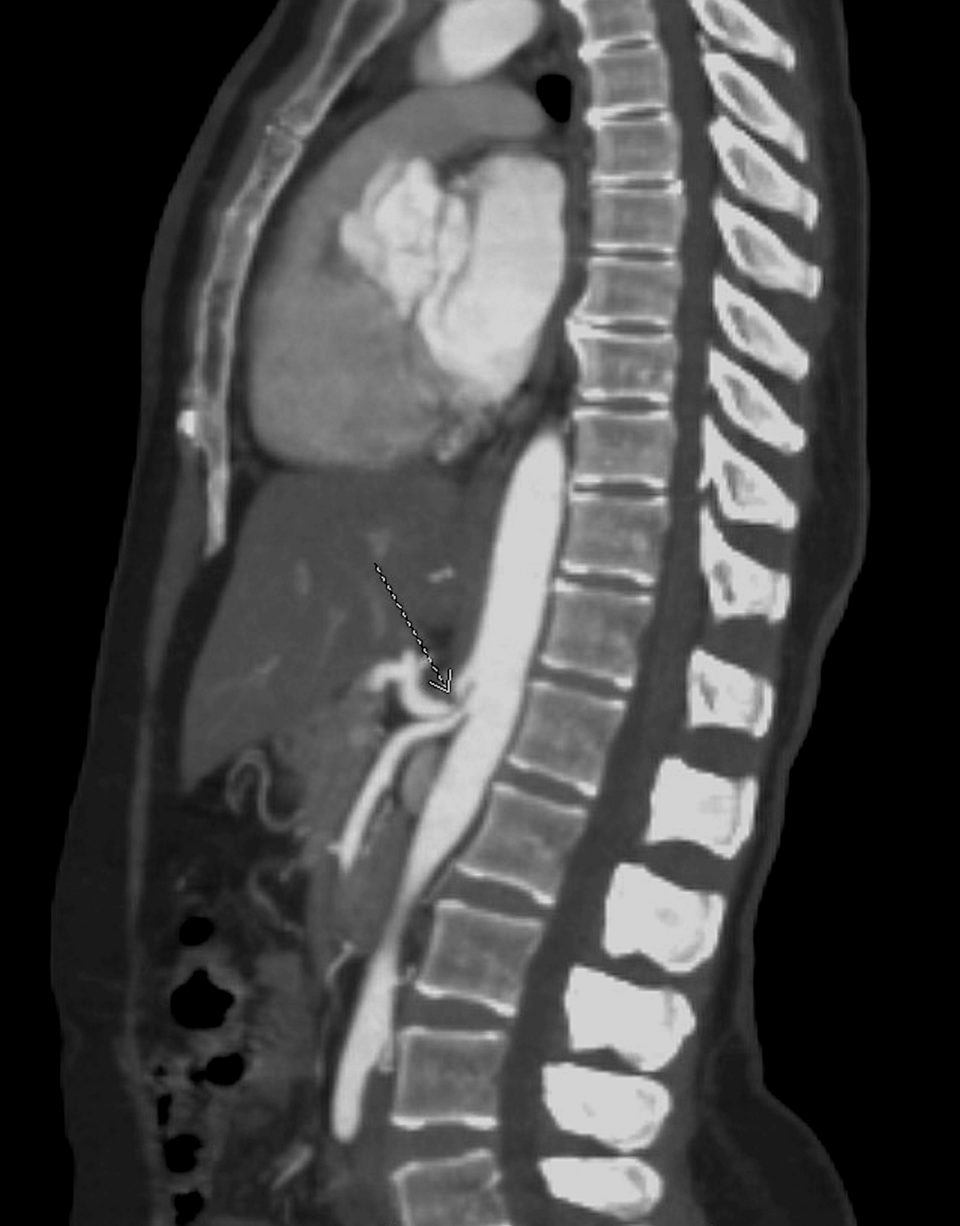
Abdominal and pelvic computed tomography angiography with contrast. The arrowhead indicates the hooked morphology of the celiac trunk, which is compatible with median arcuate ligament syndrome.
